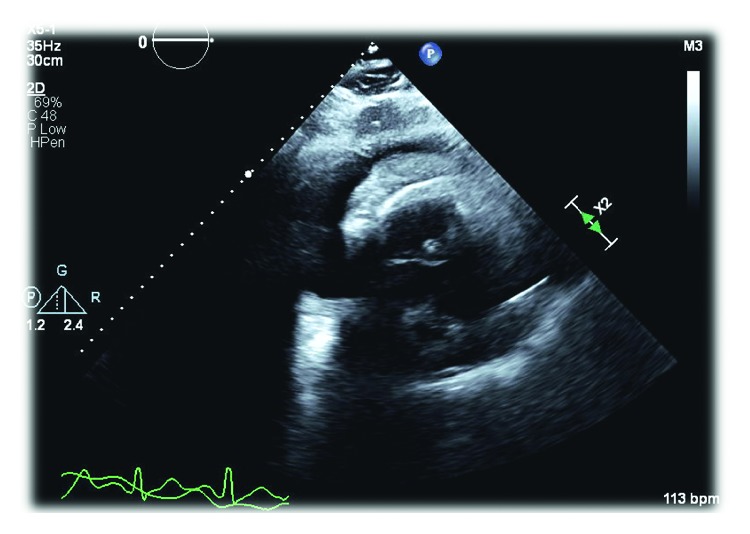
Echocardiogram showing tamponade with solid component in effusion consistent with fibropurulent exudate.
